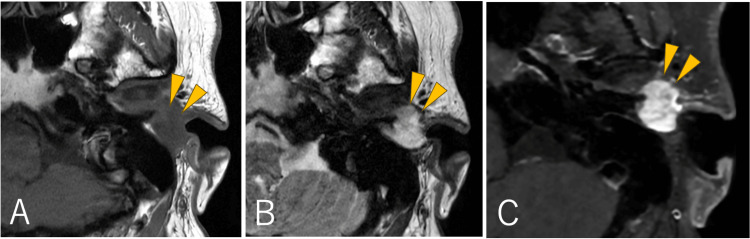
Preoperative MRI Preoperative MRI showing tumor hypointensity (arrowhead) on T1-weighted imaging (A), hyperintensity on T2-weighted imaging (arrowhead) (B), and heterogeneous enhancement on gadolinium-enhanced imaging (arrowhead) (C).
